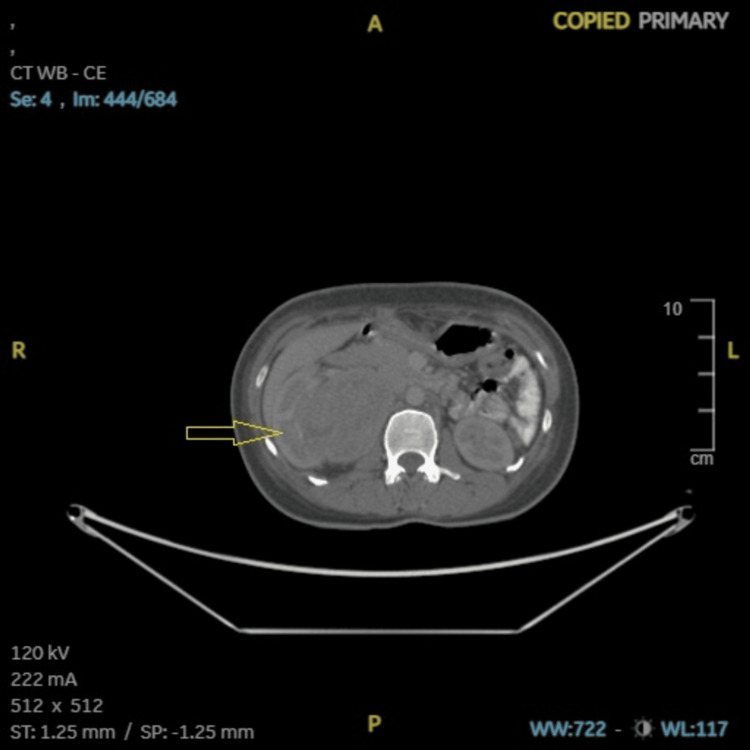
Case 2: CECT (abdomen and pelvis), a primary right renal mass measuring 6.5 × 5.5 × 6 cm (arrow). CECT: contrast-enhanced computed tomography, A: anterior, R: right, L: left, P: posterior, ST: soft tissue, SP: soft tissue window (preset), WB: whole body, WW: window width, WL: window level.
